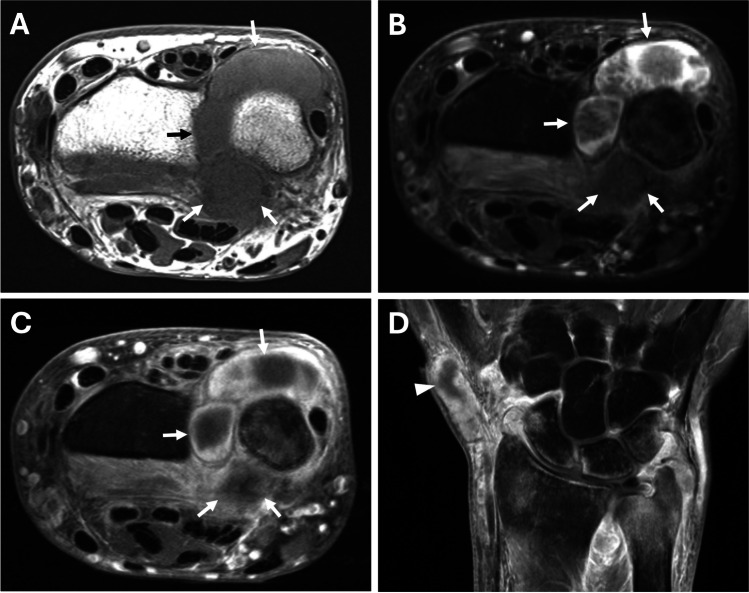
MR imaging of the left wrist. (A) Axial T1-weighted, (B) axial T2-weighted fat-suppressed, as well as (C) axial and (D) coronal post-contrast T1-weighted fat-suppressed MR images show relatively symmetric findings with the right wrist, including marked distension of the distal radioulnar joint (arrows) and extensor pollicis brevis tendon sheath (arrowhead) with variably sized, relatively hypointense and hypoenhancing masses and nodules. Polyostotic variable edema-like signal and enhancement is present throughout the wrist
